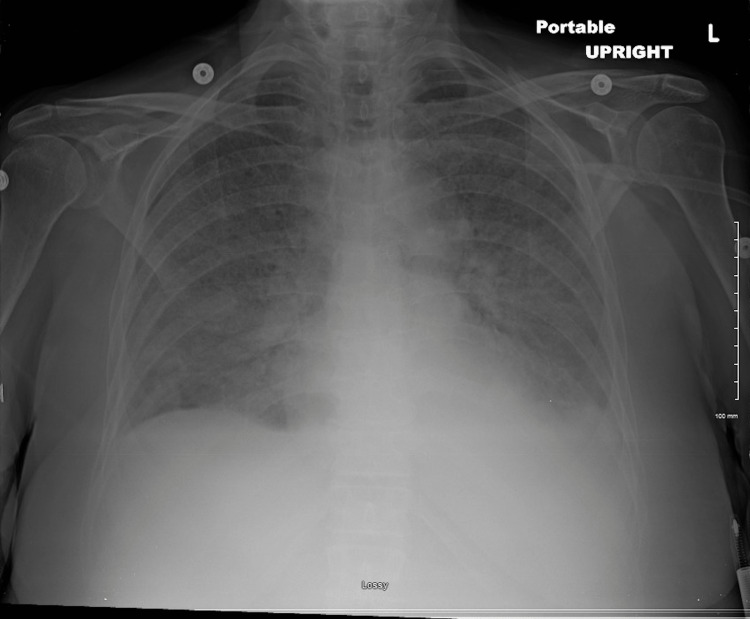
Chest x-ray on admission day 8
